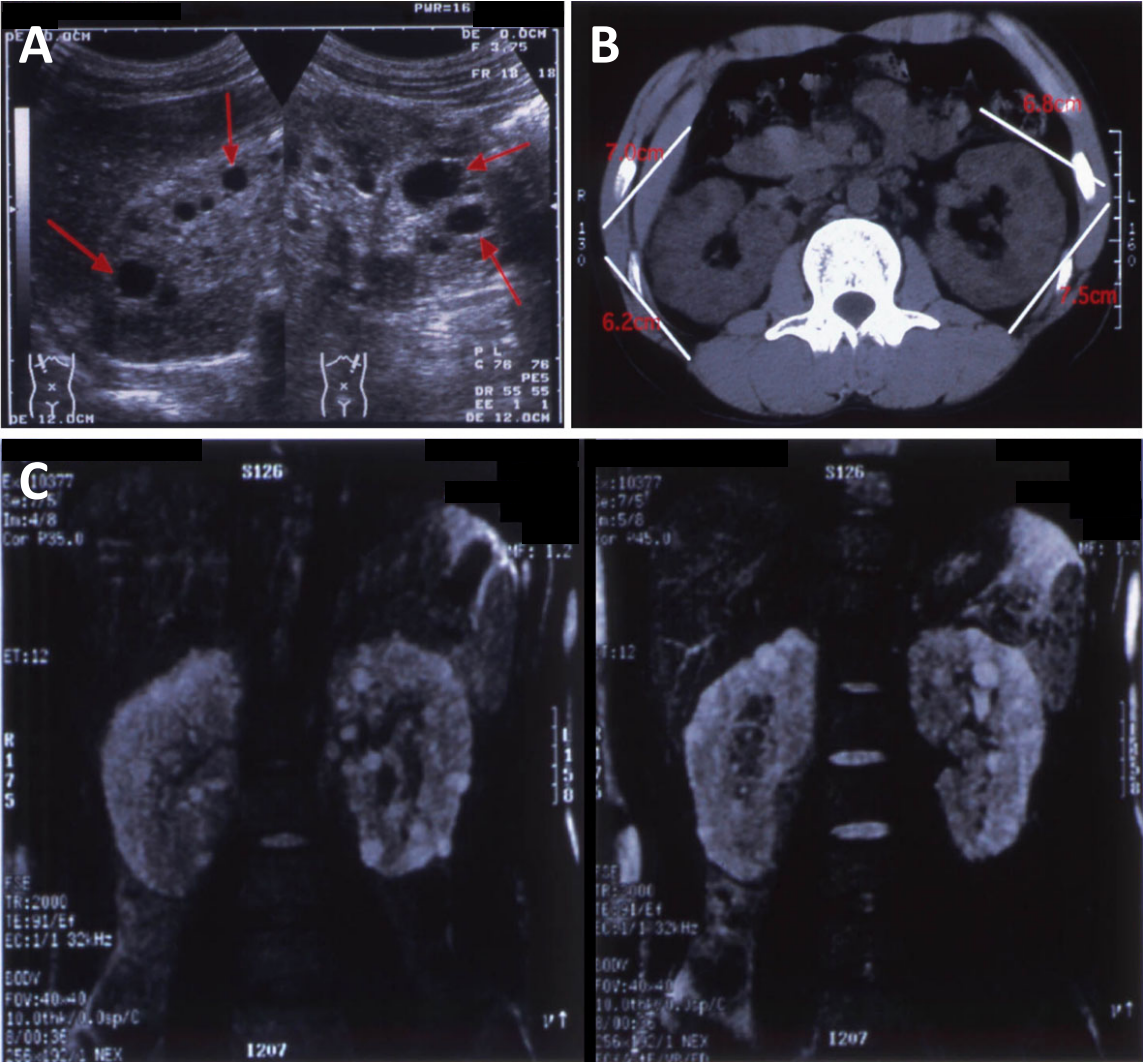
Imaging studies of the kidneys. Both kidneys contain multiple oval lesions, which are up to 20 mm in diameter, are hypoechoic, show low density on computed tomography (CT), and show high signal intensity in T2-weighted magnetic resonance imaging (MRI) (panel a: ultrasound image; panel b: CT image; panel c: T2-weighted MRI)
